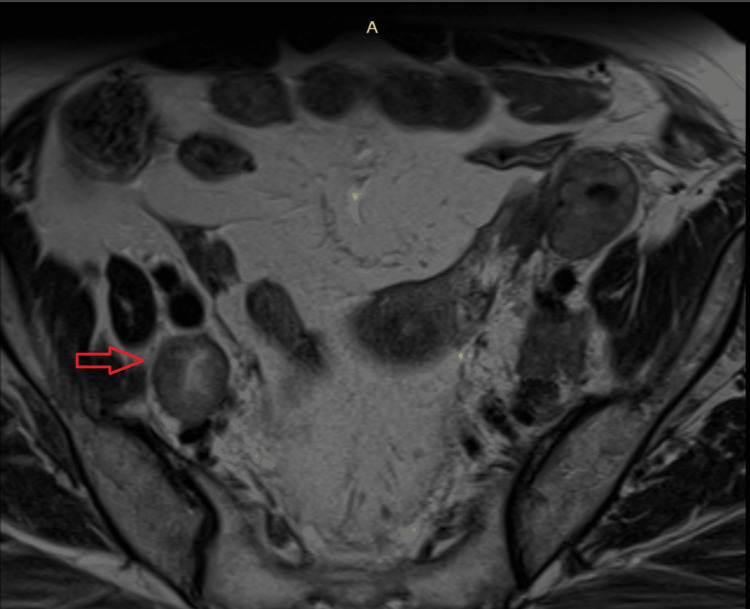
MRI image showing an involved right pelvic lymph node in an early cervical cancer patient The red arrow shows an enlarged right pelvic lymph node with a size of more than 1 cm
